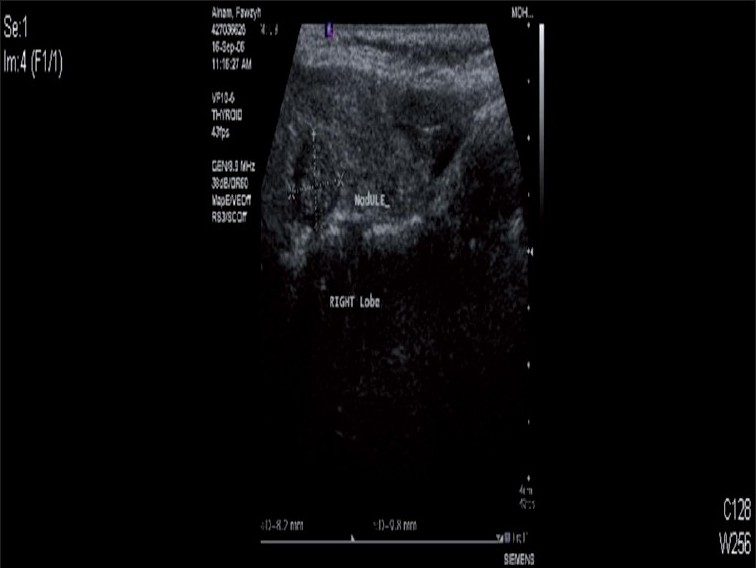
Ultrasound of the neck showing right thyroid lobe nodule
